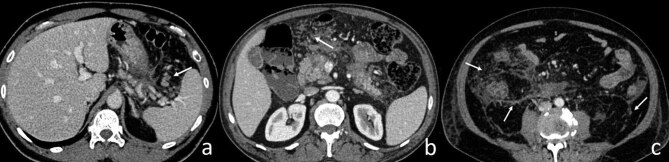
Peripancreatic FND (arrow) (a), anterior pararenal, and mesenteric FND (arrow) (b) are seen on the axial contrast-enhanced abdomen CT images of two different patients with necrotizing pancreatitis. FND localized at the paracolic area, lateroconal fascia, beyond Gerota’s fascia, and posterior renal fascia (arrows) are seen on the axial contrast-enhanced abdomen CT image (c).
